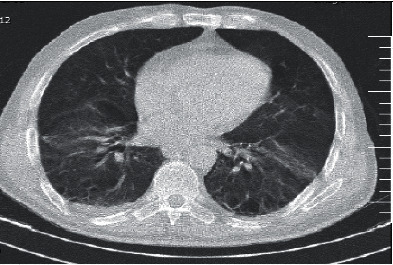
Male patient's chest CT.
